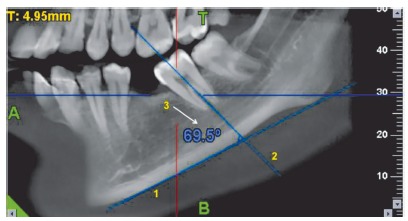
Calculation of mesiodistal angulation of the second molar on an 11×5-cm CBCT section of the mandible.
